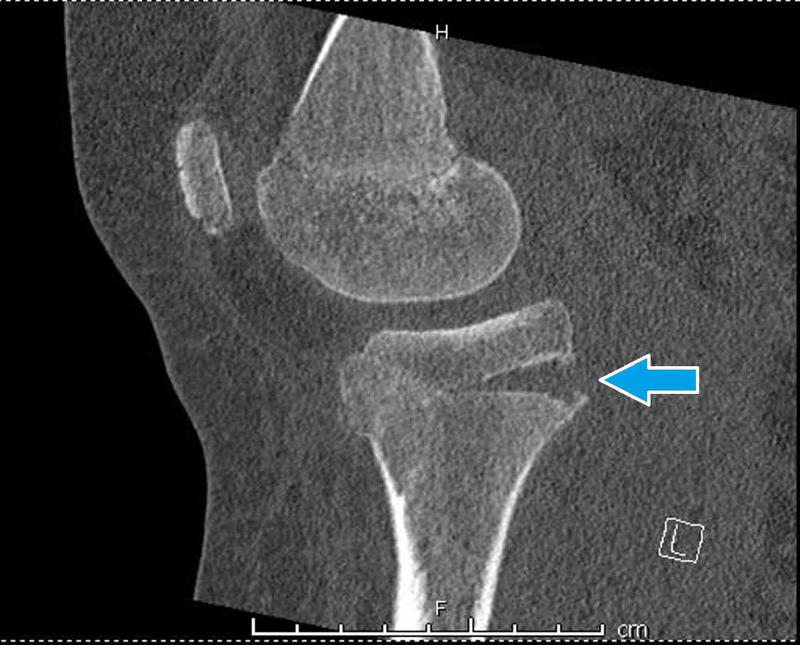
CT of left knee; sagittal view of medial femoral condyle and tibia
